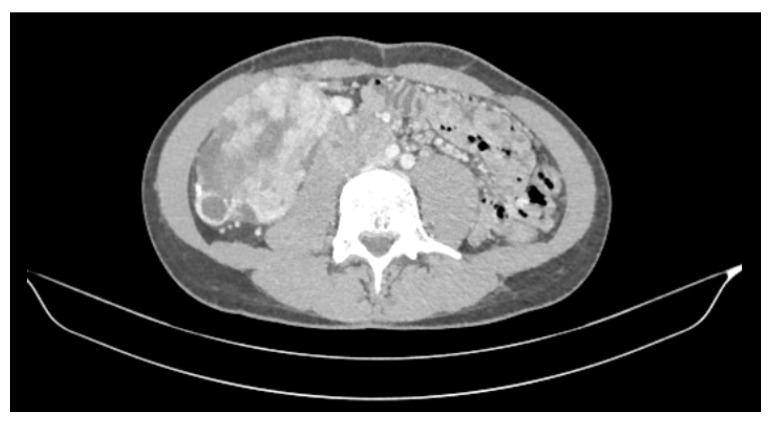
CT at diagnosis. Mass at the upper pole of the right kidney.
